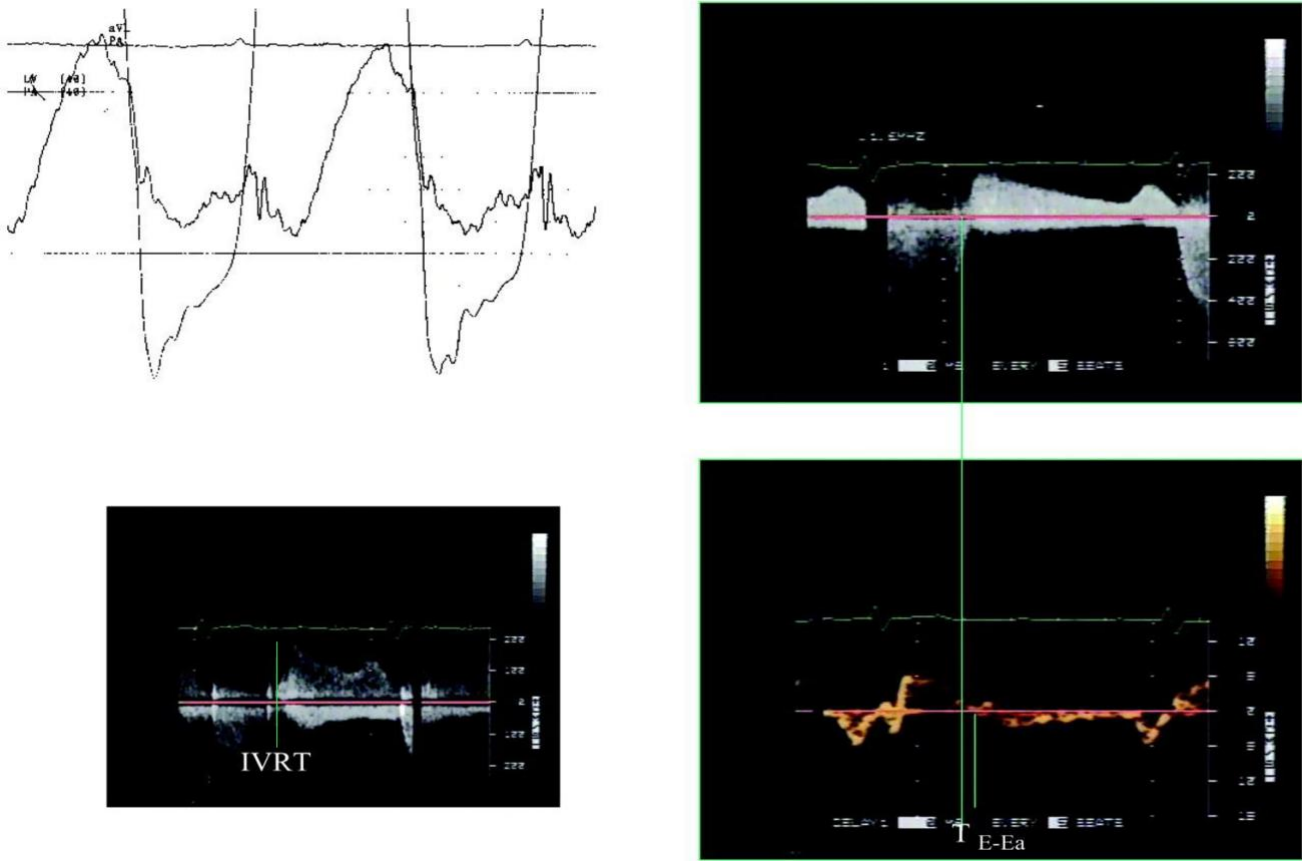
Pressure and Doppler signals from patient with combined mitral regurgitation and mitral stenosis. Upper left, LAP and LV pressure tracings; lower line marks 20 mmHg, and upper line, 40 mmHg. Upper right, mitral inflow. Lower left, IVRT (interval between end of aortic flow at closing click and green line marking onset of mitral inflow). Lower right, mitral annulus tissue Doppler recording at septal site of mitral annulus. Note prominent v wave in LAP. Mean LAP was 31 mmHg. Mitral valve area was calculated at 1 cm^2^. IVRT was 56 ms, and T_E–e′_ was 56 ms, giving an IVRT/T_E–e′_ ratio of 1. Ea in the lower right panel represents e′. Modified from Diwan *et al*.^51^
